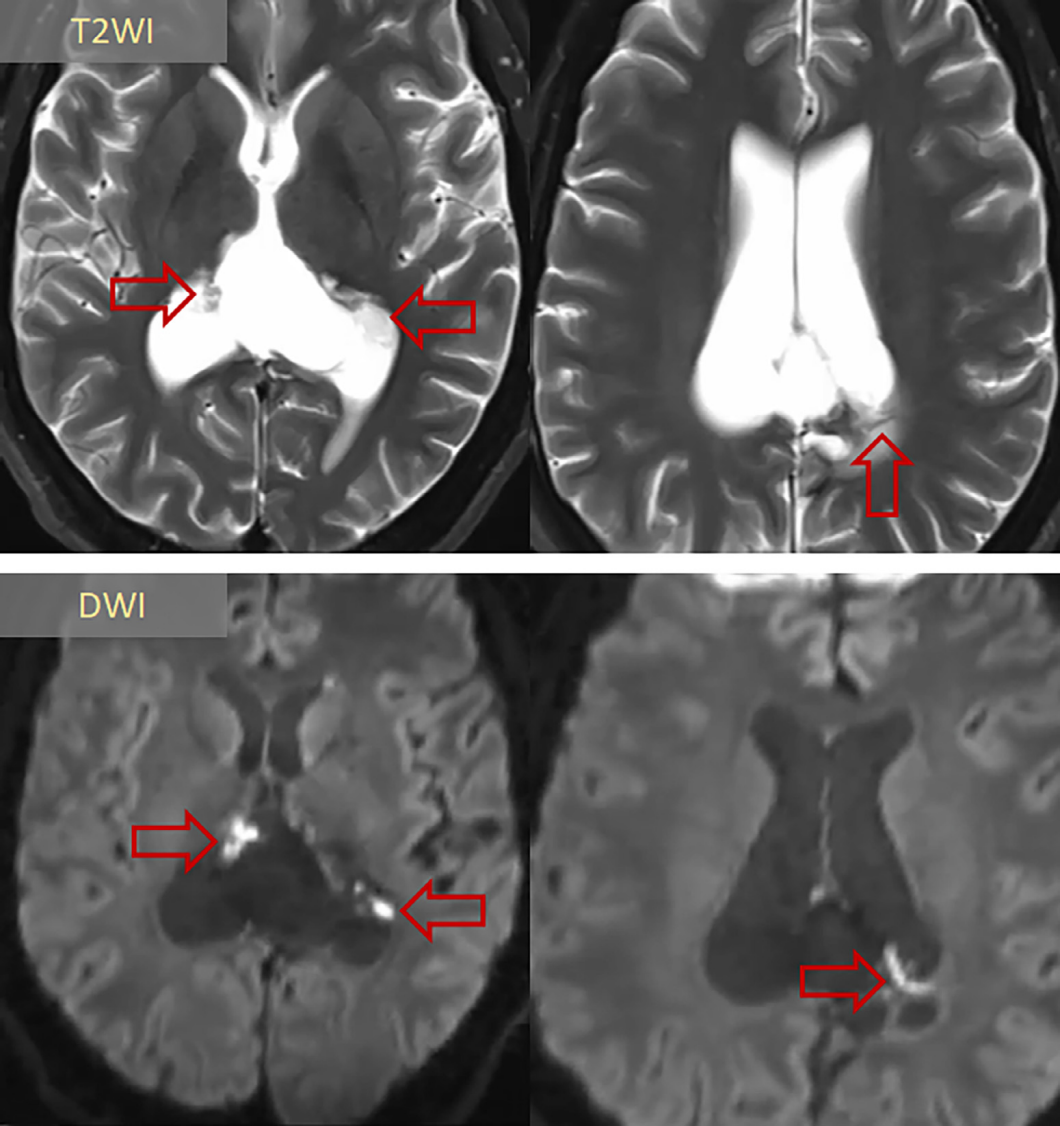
Three-month postoperative MRI surveillance following near-total resection (>95% tumor volume reduction). (A) T2WI and (B) DWI demonstrate minimal residual tumor remnants (*arrows*) deliberately preserved beneath the ependymal layer to prevent neurological morbidity, with no evidence of postoperative complications. *T2WI: T2-weighted imaging; DWI: diffusion-weighted imaging.*
